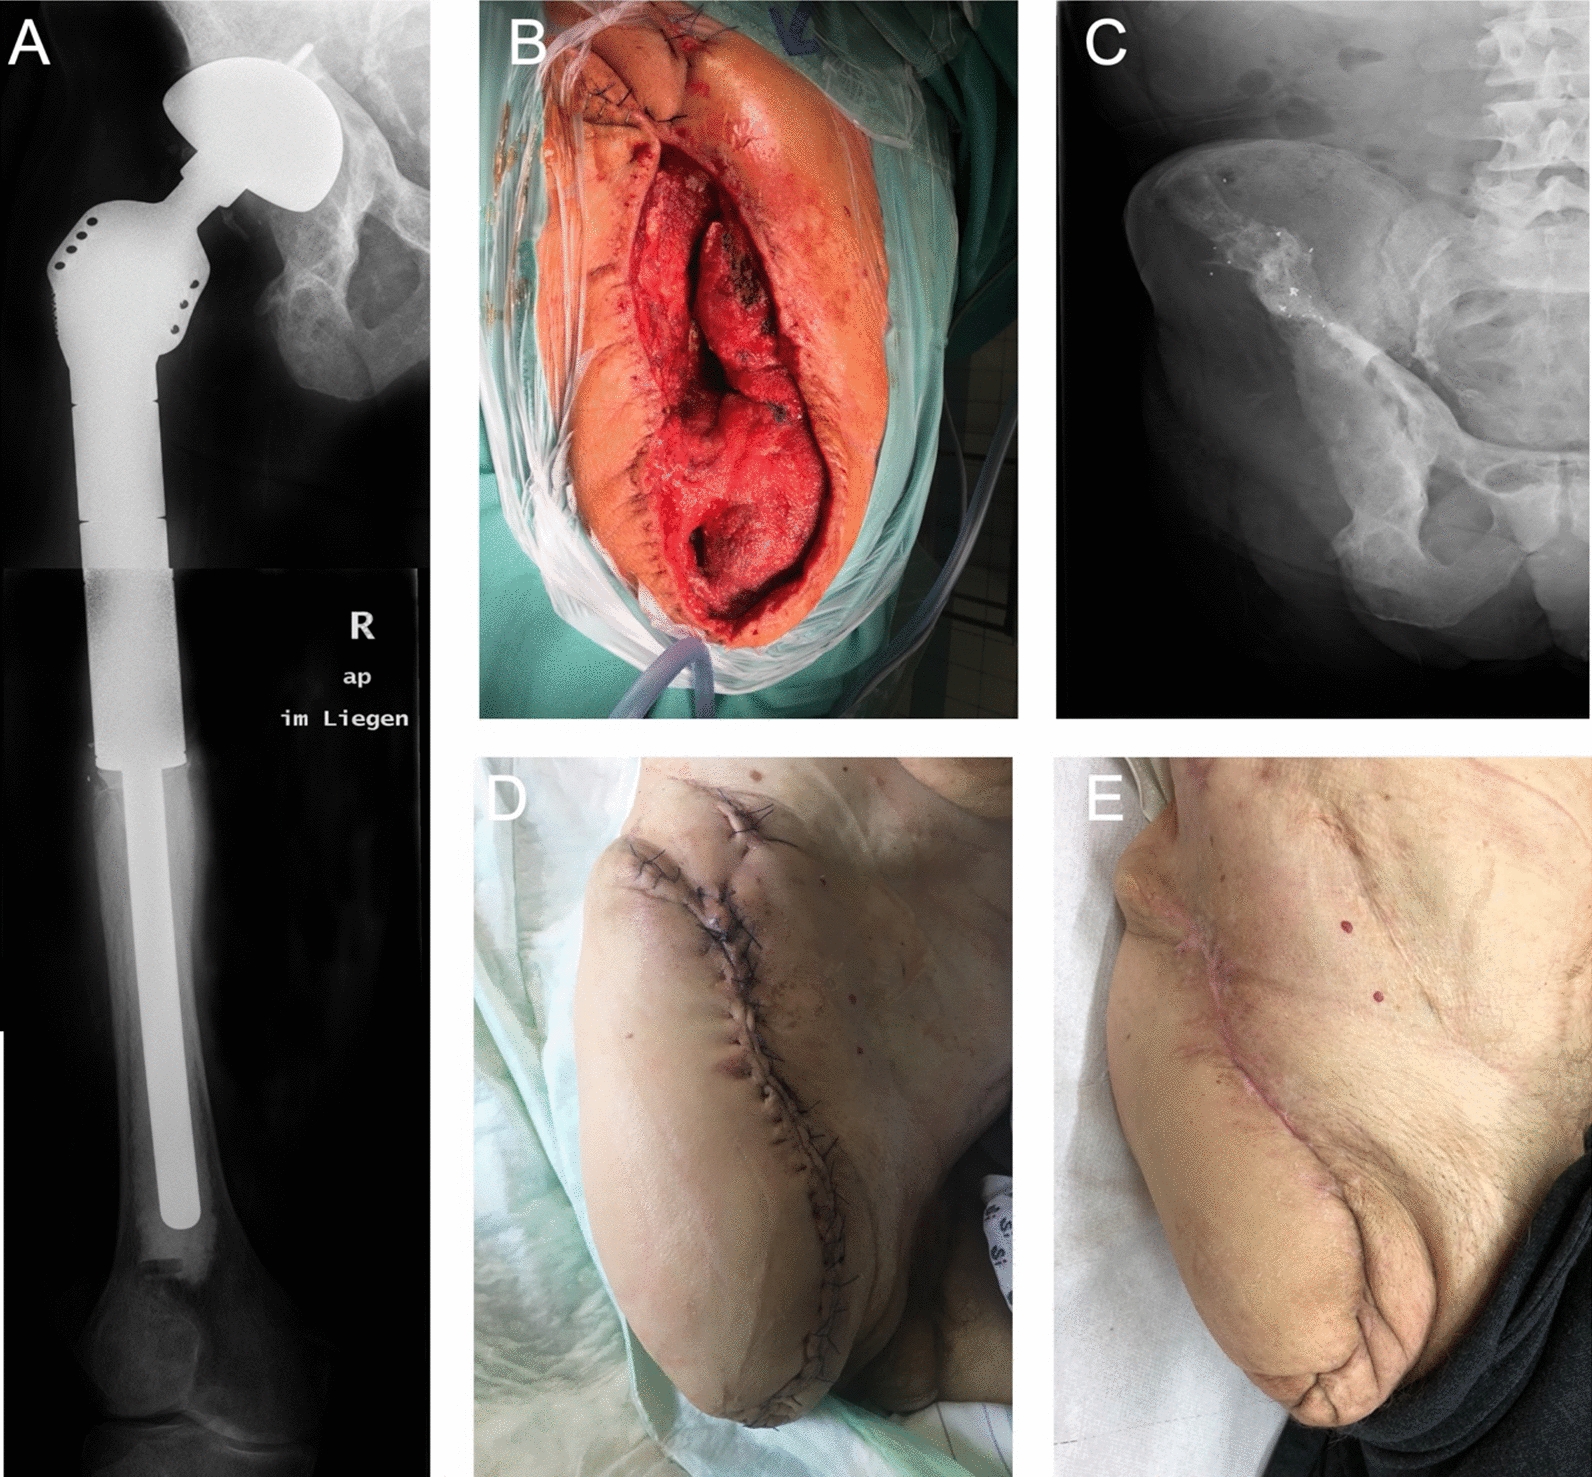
An example of the course of case 3. A shows the implanted endoprosthesis, B shows the final intraoperative result, C postoperative X-ray of the amputation stump, D wound healing during the course and E the healed stump 2 years postoperatively
